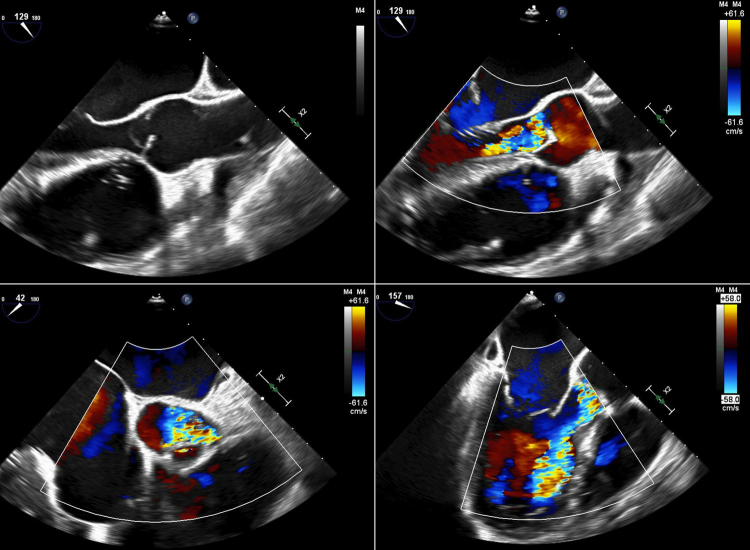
Representative transesophageal echo images showing severe aortic insufficiency with prolapse of the noncoronary cusp and malcoaptation of the right and left coronary cusps.
